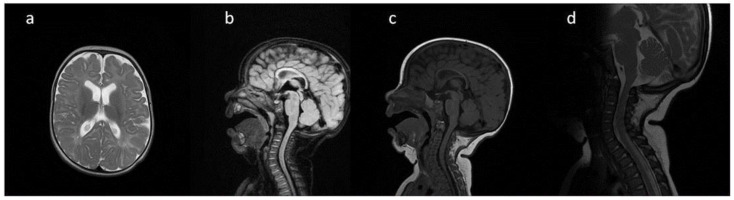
Brain and cervical spine MRI. Patient 1. (younger sister) (a) axial T2; (b) sagittal T2 FLAIR, (c) sagittal T1; (d) sagittal spinal T2. Findings: delayed myelination, slight skiboot sella turcica shape; suspicion of dens hypoplasia—for observation (age 4 mo).
